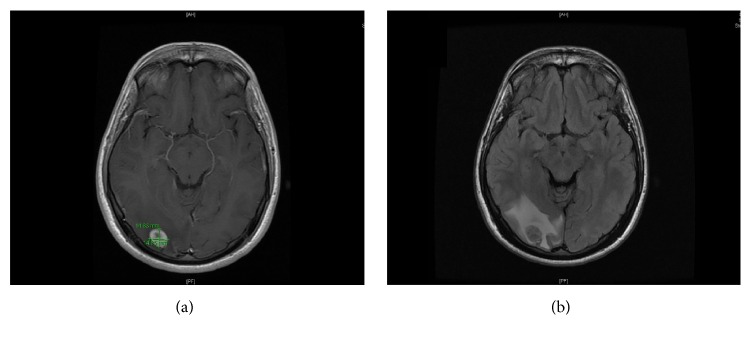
Brain MRI with contrast showed right parieto-occipital cortical and subcortical mass lesion measuring about 1.5 x 1.5 cm.
